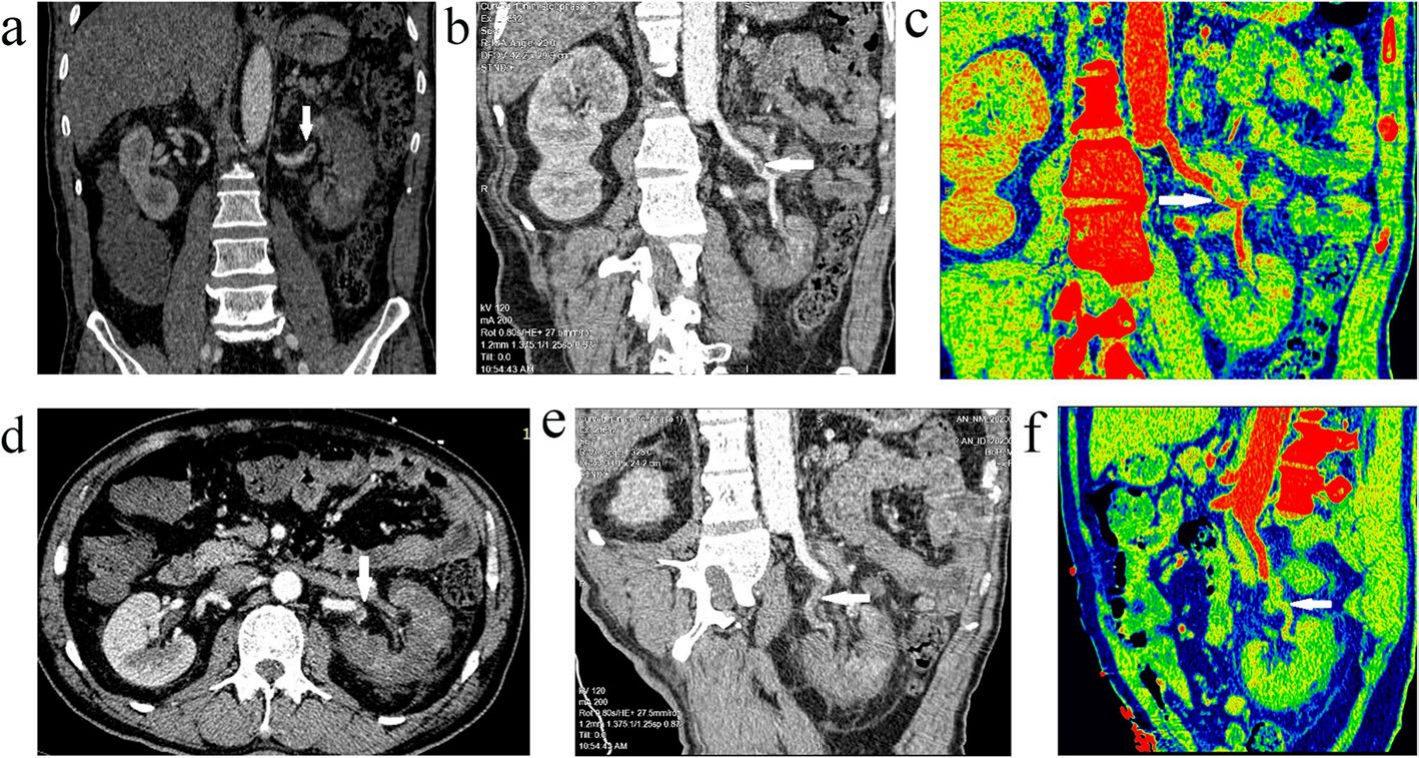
Contrast-enhanced CT indicates the filling defect of the main trunk (a, b, c, white arrow) and its posterior branches (d, e, f, white arrow) of the left renal artery
